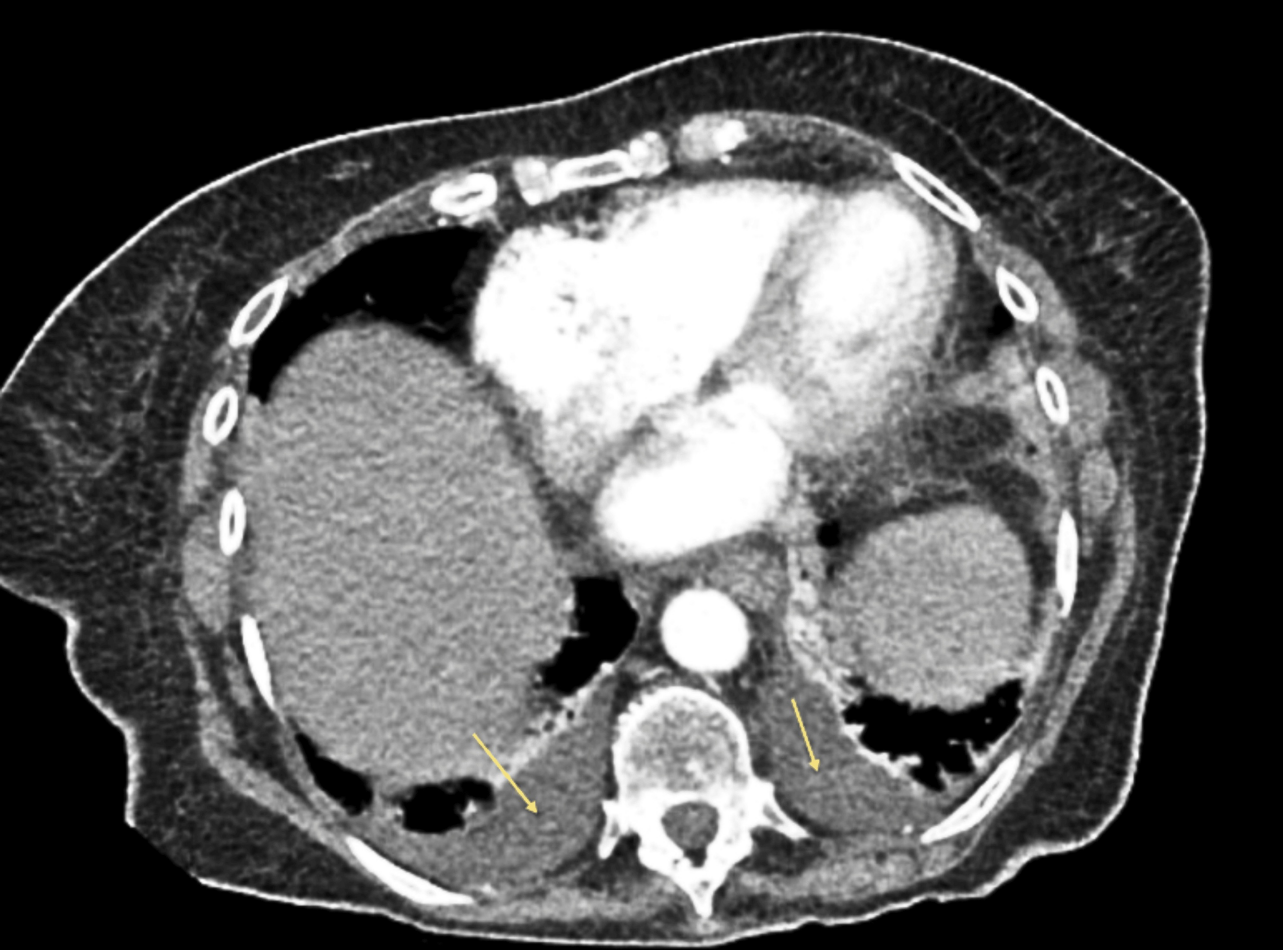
CT abdomen/pelvis with IV contrast (Axial view) Mild-moderate bilateral pleural effusions, yellow arrows
